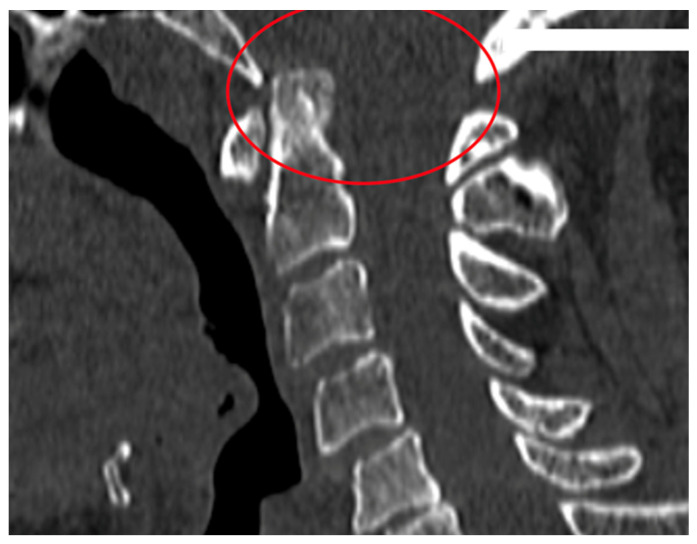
A 61-year-old patient with RA and CrS instability. Cervical CT.
